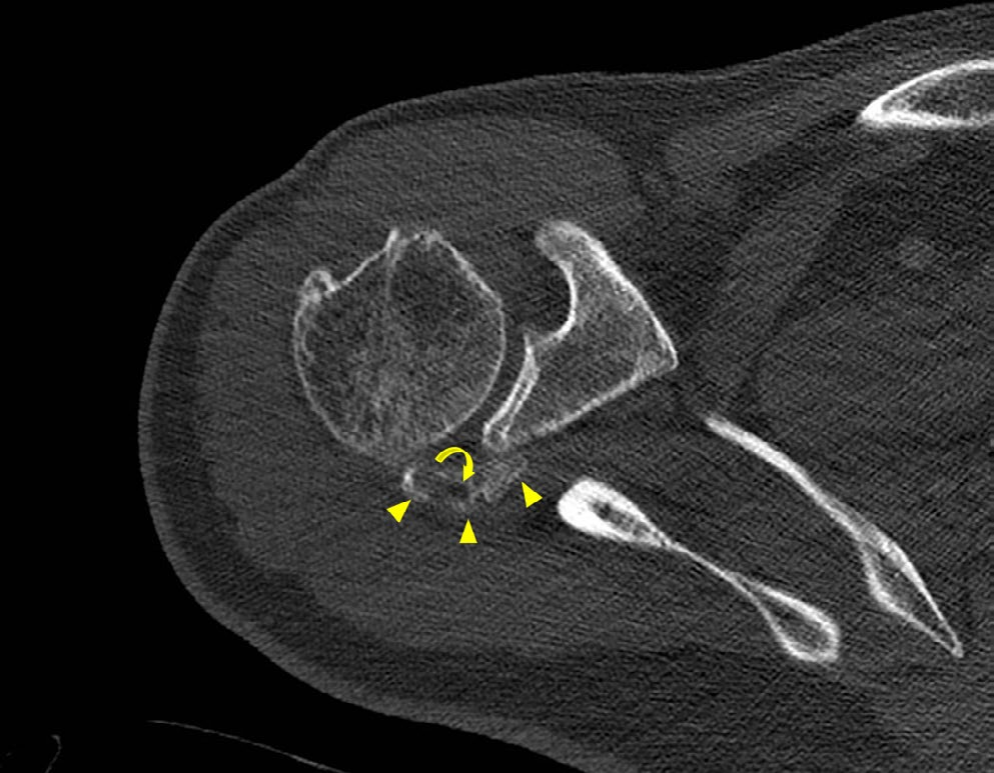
Axial computed tomography image showing an ossified mass localized in the infraspinatus muscle (yellow arrowheads). The center (curved arrow) appears with lower attenuation than the periphery of the lesion due to less mineralization
